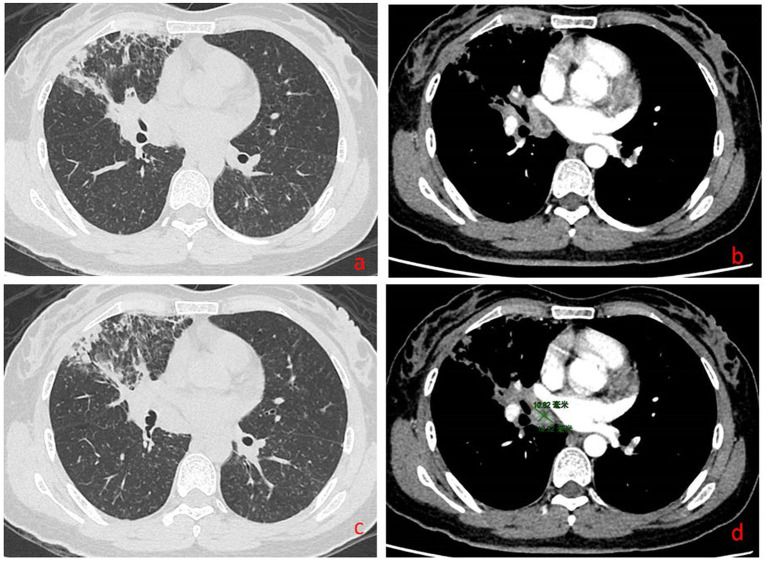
Chest CT (September 27, 2022). (a-d) Soft tissue density shadow adjacent to the right middle lobe hilum with corresponding bronchial narrowing. Contrast‑enhanced and angiographic imaging show abnormal enhancement and vascular compression. Multiple lesions and enlarged lymph nodes are seen in both lungs. Bronchoscopy confirmed *Aspergillus fumigatus* complex.
